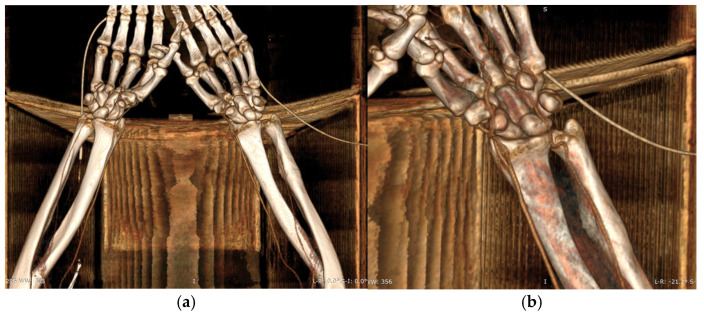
(a,b). CTA of upper limbs (a). CTA of the left upper limb (b).
